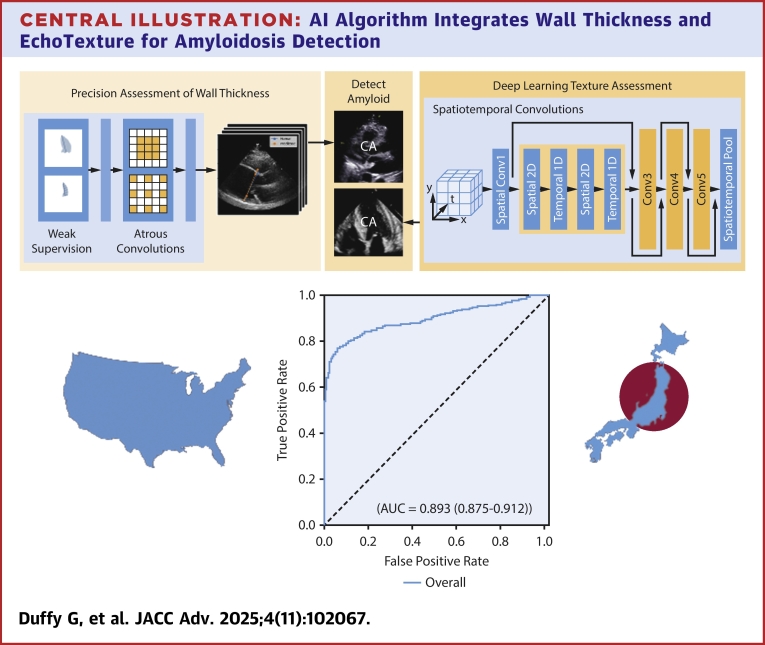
AI Algorithm Integrates Wall Thickness and EchoTexture for Amyloidosis Detection A fully automated computer vision algorithm identifies key features from the parasternal long axis and apical-4-chamber echocardiogram videos to identify cardiac amyloidosis from visual features. This algorithm was evaluated across five geographically and ethnically diverse cohorts across the United States of America and Japan to show strong generalizable performance across a range of ultrasound vendors and patient demographics. AUC = area under the receiver operating characteristic curve; CA = cardiac amyloidosis.
